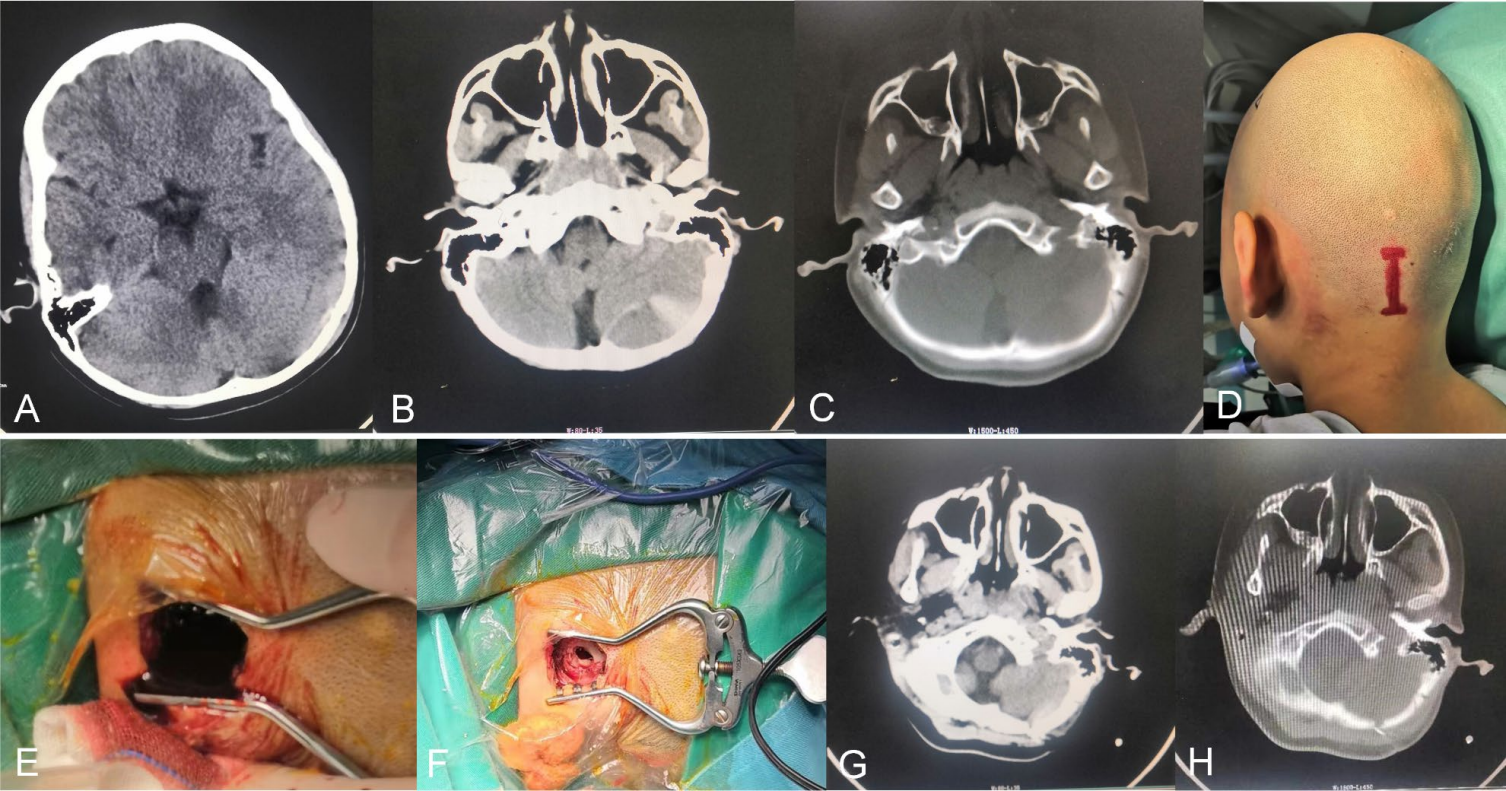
A 9-year-old patient with LEDH. A Occipital skin swelling and a small amount of epidural hematoma were found 3 h after injury. B The size of the hematoma was increased in the cerebellar hemisphere 7 h after injury. C The fracture line of the occipital bone was observed through the bone window. D Intraoperative incision. E Dark-red hematoma flows out after drilling. F After the hematoma had flown out, no CSF came out of the bone hole. G, H Postoperative CT scan reexamination showing hematoma elimination
